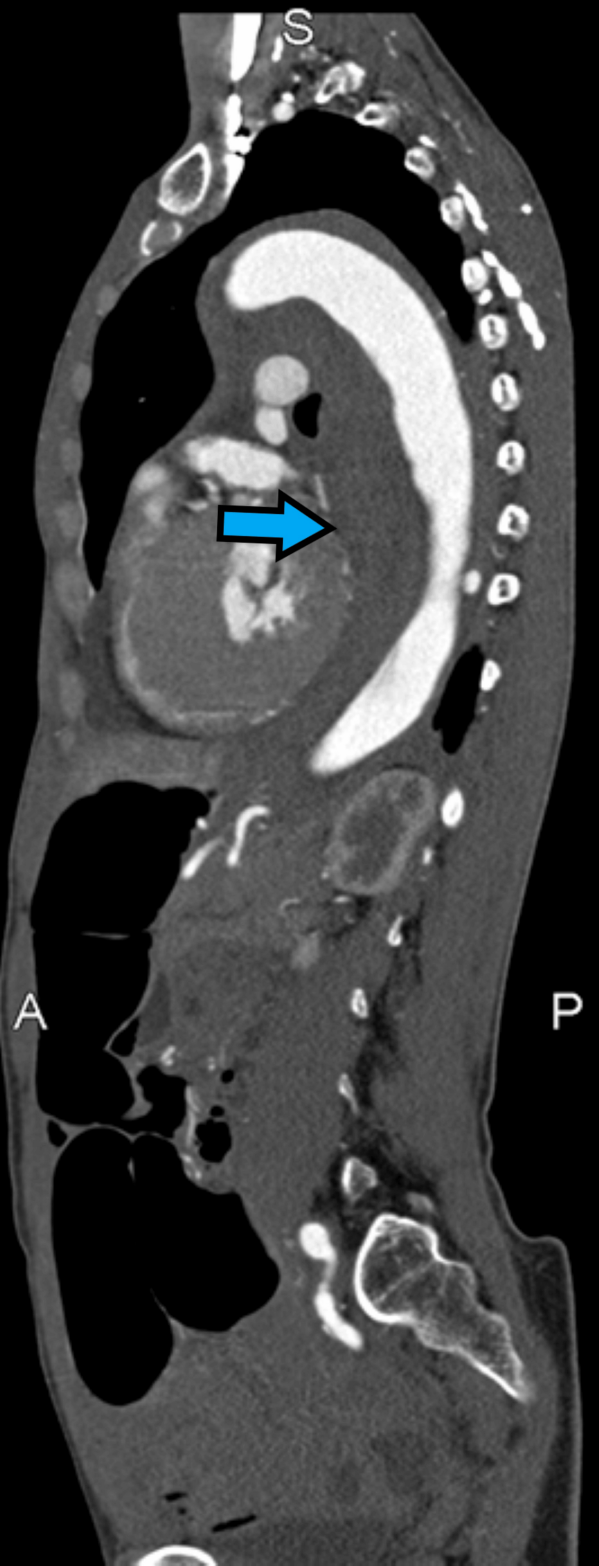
Computed tomography angiogram, sagittal plane, demonstrating a large hematoma within the false lumen of a type B aortic dissection as well as dilated loops of bowel.
